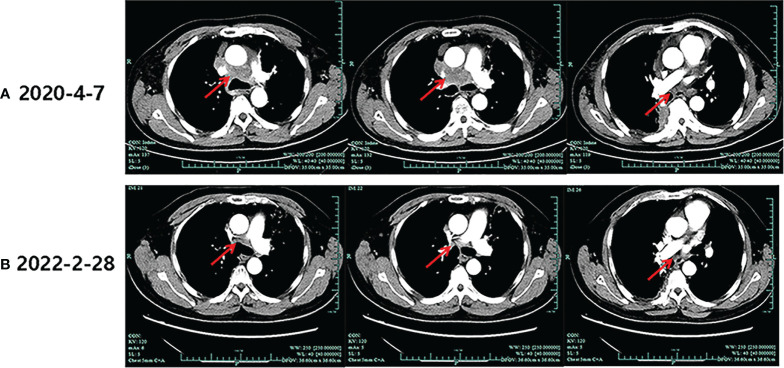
Imaging evolution of the patient. (A) The baseline computed tomography scan of alectinib treatment. (B) Computed tomography scan of the last follow-up of alectinib treatment.
